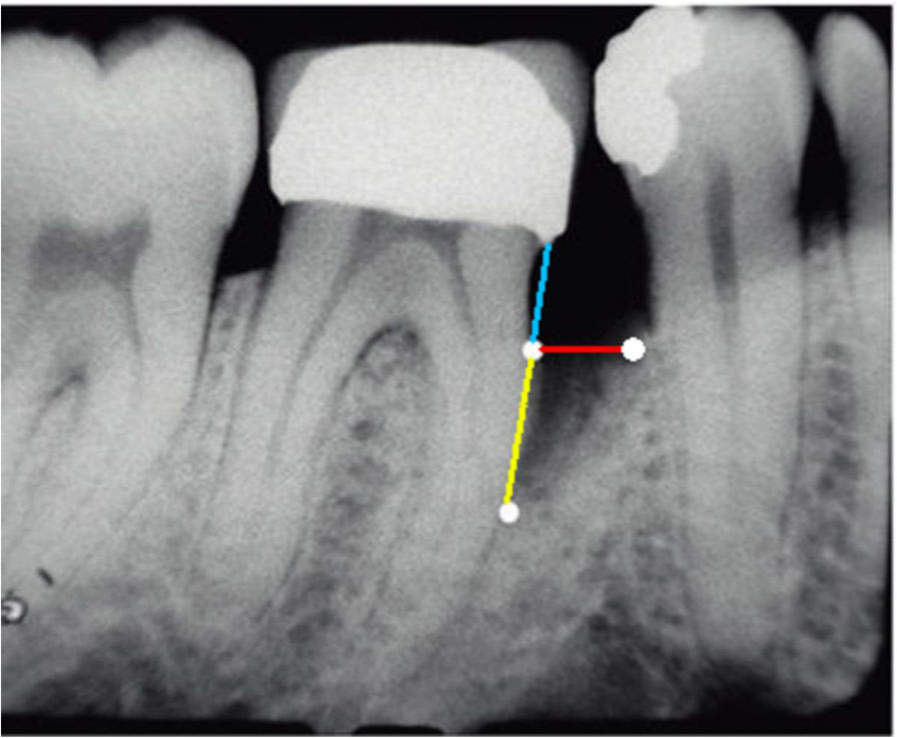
(Adapted from Parashis et al., 2012). Radiographic parameters evaluated in intrabony defect. Blue: BCL; yellow: VC; red: HC; blue+yellow: BDL
